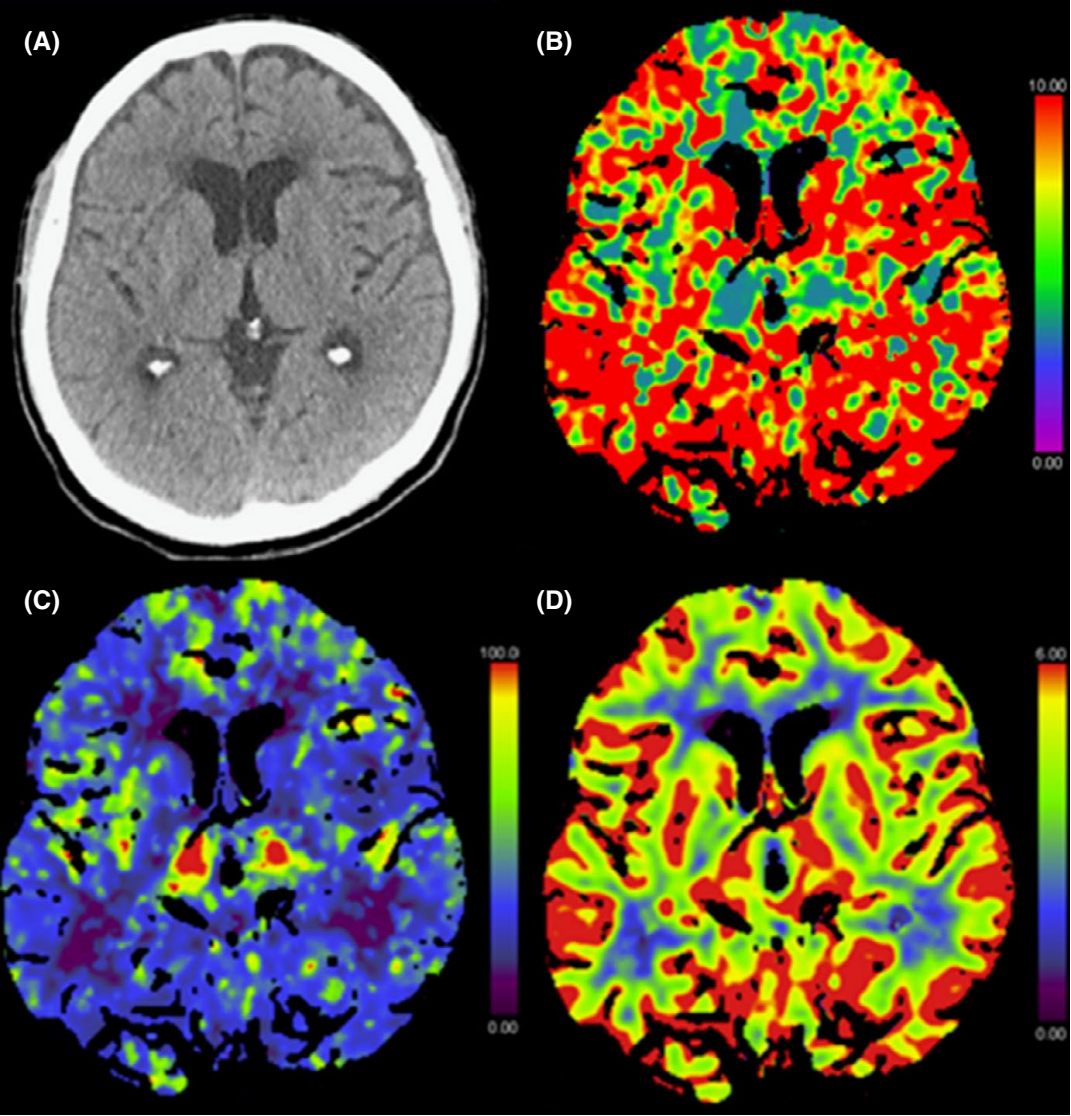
Initial axial non‐enhanced computed tomography (CT) (A) showing normal parenchyma with no evidence of swelling or ischemic changes. CT perfusion showing prolonged mean transit time (MTT) (B) with global cortical hypoperfusion including the basal ganglia in the cerebral blood flow map (C), but no abnormalities in the cerebral blood volume map (D)
